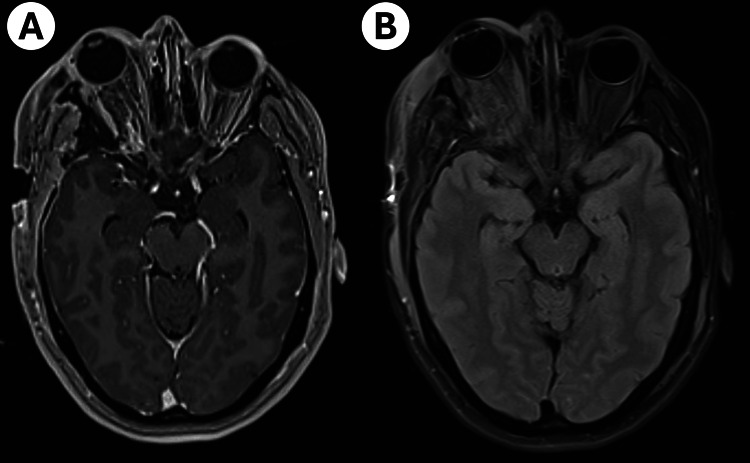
MRI images at 12-month follow-up A. Postoperative T1 with Gadolinium contrast demonstrates removal of the intraconal and intracranial components with persistent contrast enhancement. The optic nerve remains intact throughout its trajectory. B. T2 TIRM sequence reveals edema affecting the intraorbital muscles. TIRM, Turbo Inversion Recovery Magnitude.
